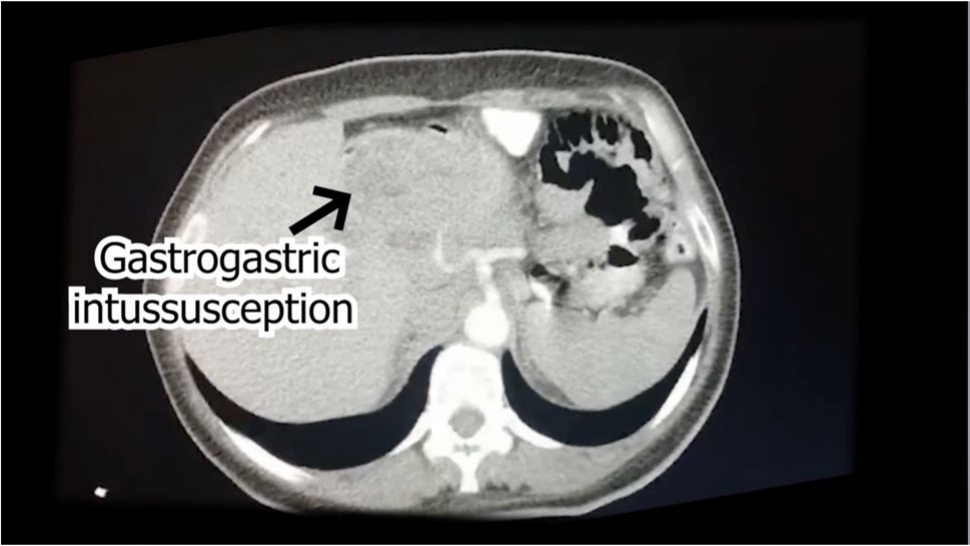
CT scans of gastrogastric intussusception
